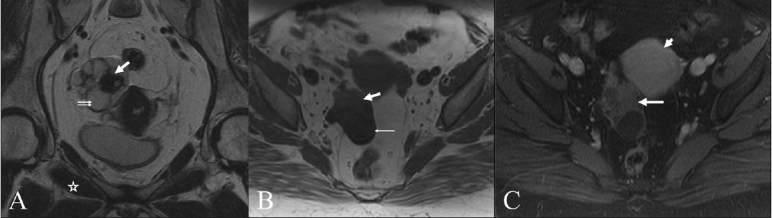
(A–C) Oblique coronal T2W (A), axial T1W (B) and axial postcontrast, fat-saturated T1W (C) MRI images show a complex right adnexal mass with cystic and solid components. The cystic component shows high-T2 signal (double arrow in A) and low-T1 signal (thin arrow in B), while the solid, fibrous component shows low-T2 signal (thick arrow in a) and intermediate T1 signal (thick arrow in B), isointense to skeletal muscle (star in A). Mild enhancement (long arrow in C) relative to the adjacent uterine myometrium (short arrow in C) is seen
